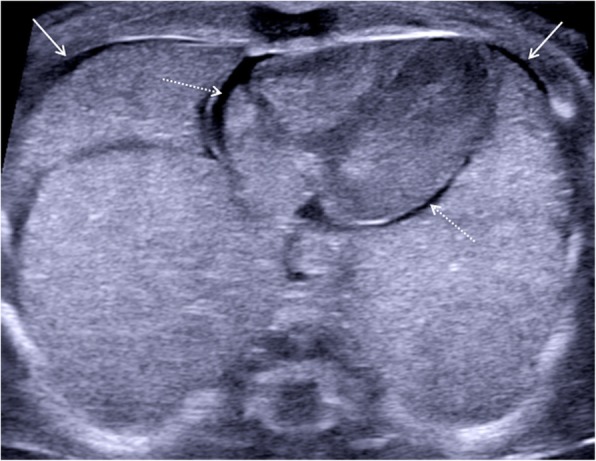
Transverse postmortem ultrasound image through the chest in a 19-week gestational-aged foetus, obtained 8 days after death showing normal postmortem fluid accumulation within the pleural spaces (solid white arrows) and pericardium (dotted arrows) bilaterally. These are commonly seen and described as ‘postmortem changes’ and should not alarm the ultrasound operator
